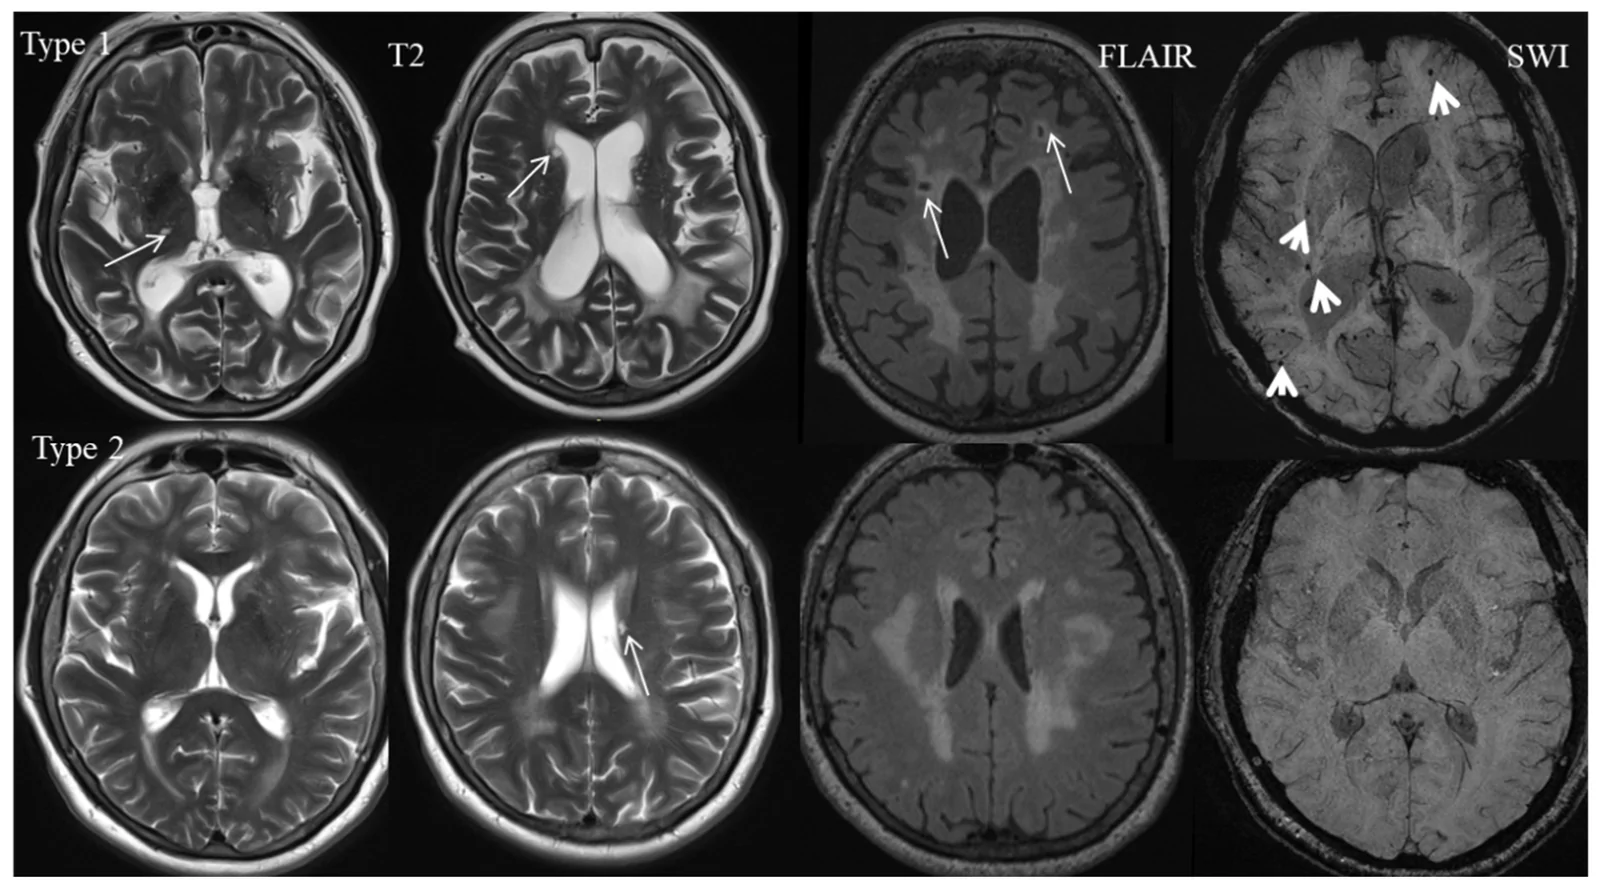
Examples of brain MRI in patients with structural changes characteristic of MRI Type 1 (upper row) and MRI Type 2 (lower row). Thin arrows—lacunes; short arrows—cerebral microbleeds.
